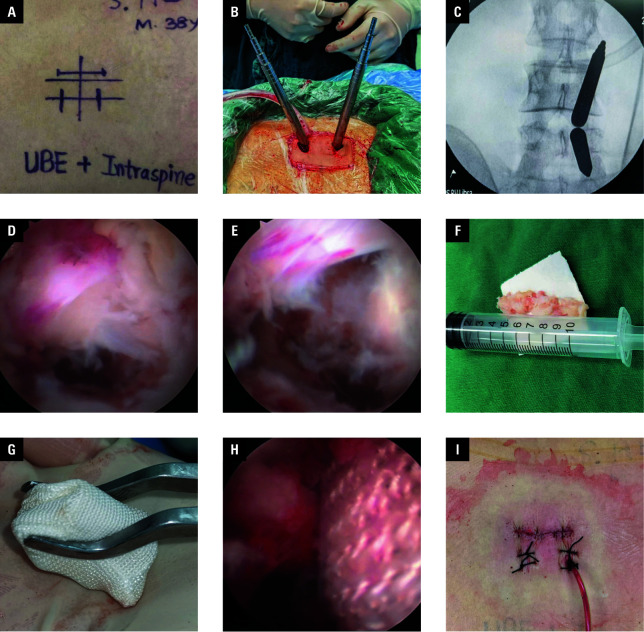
A – design of the incision; B – intraoperative picture showing 2 serial dilators utilized to progressively expand and bluntly separate the soft tissue; C – final position of the 2 dilators directed toward the lower lateral edge of the interlaminar space on X-ray fluoroscopy; D – the extruded intervertebral disc nucleus (arrow) compressing the L5 nerve root (asterisk); E – successful decompression of the nerve root (asterisk); F – the large extruded intervertebral disc nucleus in vitro (arrow); G – the IntraSPINE dynamic stabilizing device in vitro (arrow); H – the IntraSPINE dynamic stabilizing device (arrow) on endoscopy; I – suturing of the incisions and placement of a drainage tube
